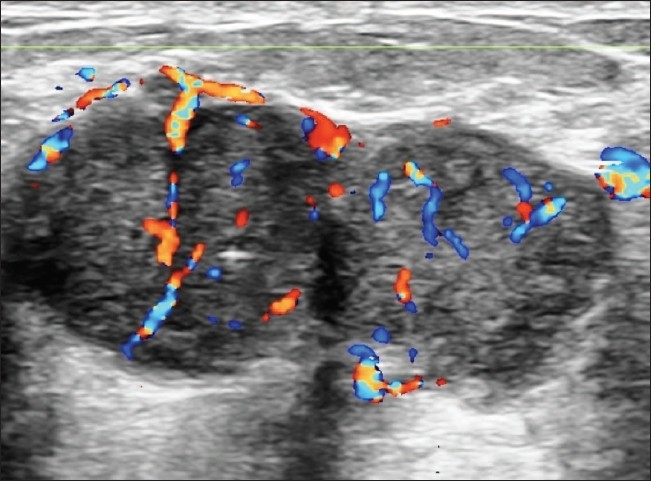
Malignant lesion. A smooth margin and homogenous echotexture suggest a category 3 lesion. Color Doppler reveals irregularly branching neovascularity
